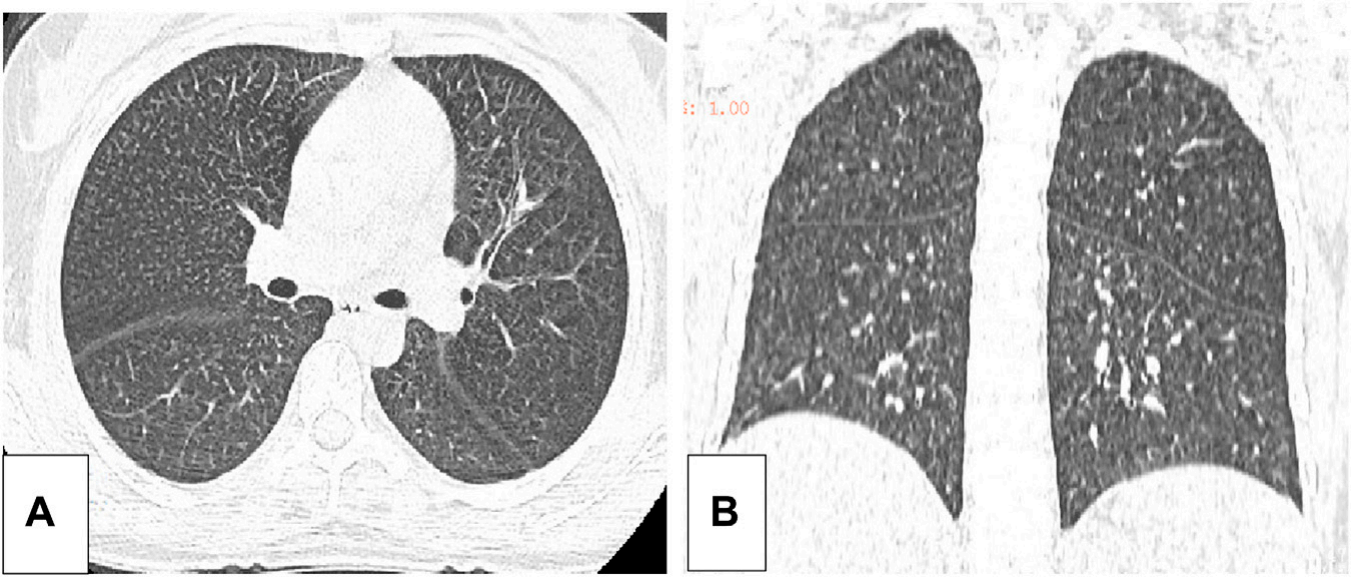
(A) The axial position of the high resolution thoracic CT of the patient. (B) The coronal plane of the high resolution CT of the patient.
